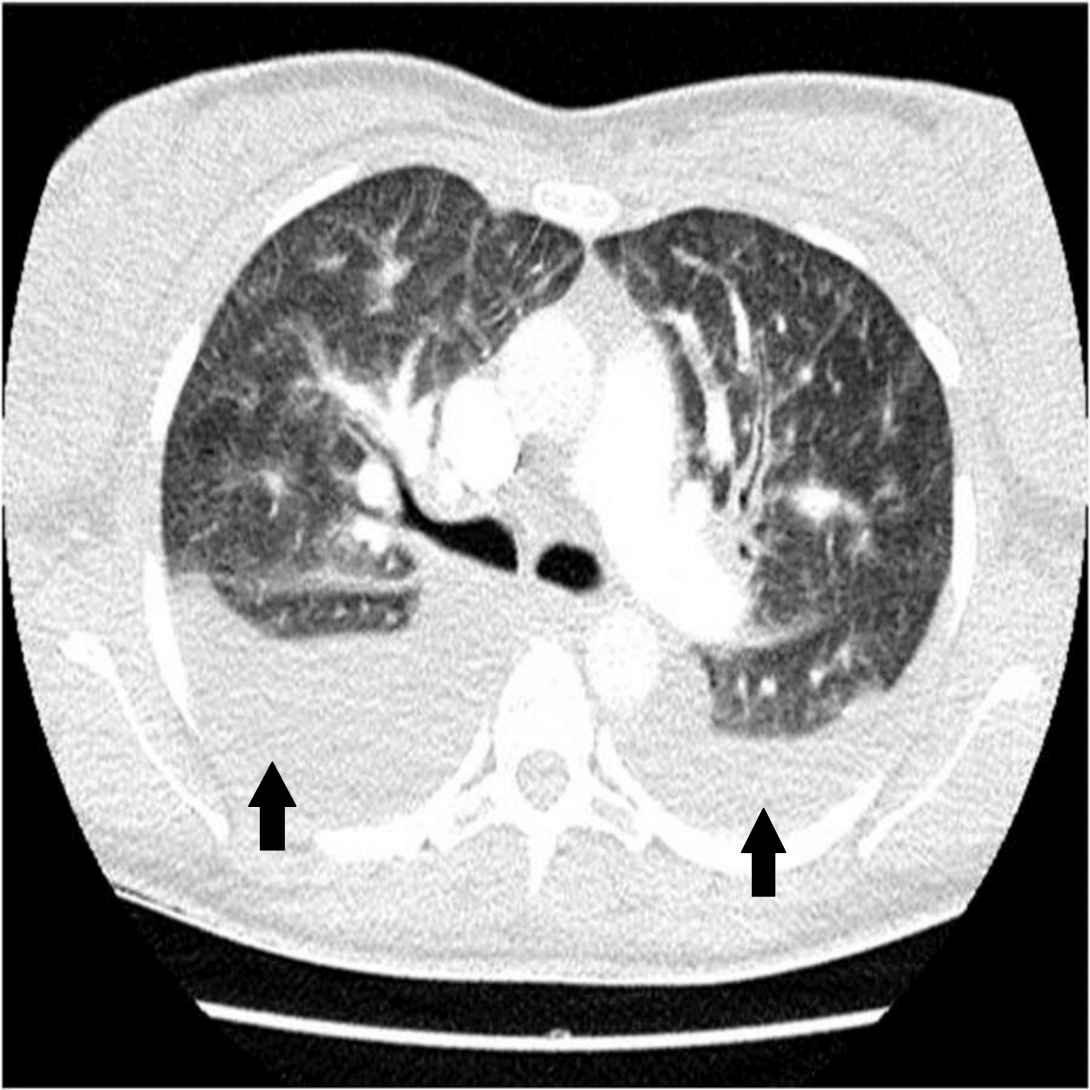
Computed tomography pulmonary angiography (axial view) showing significant pleural effusions (black arrows) bilaterally.
